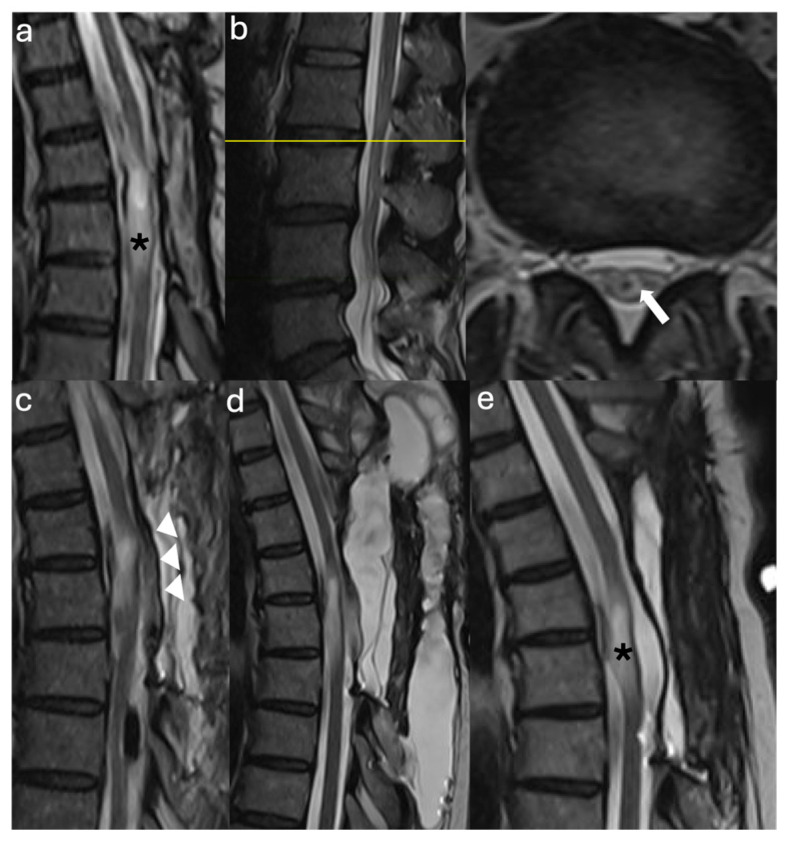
Radiographic Images Case 5: (a) Preoperative sagittal thoracic MRI T2 sequence shows syringohydromyelia associated with myelomalacia (asterisk) extending from T4–T6 at the prior T3–T5 laminectomy site for prior syrinx decompression. (b) Preoperative sagittal and axial lumbar MRI T2 sequences show a low-lying conus at the mid-L3 vertebral level. The conus tip is seen at the axial sequence at the upper L3 level (yellow bar, arrow). (c) Early postoperative sagittal thoracic MRI T2 sequence shows a significantly smaller thoracic syrinx with stable myelomalacia, expansile duraplasty (arrowheads). (d) One-month postoperative sagittal thoracic MRI T2 sequence shows a dorsal T3–T6, 4.7 × 3.4 × 11.1 cm sized pseudomeningocele. (e) Sagittal thoracic MRI T2 sequence after two duraplasty revisions shows no residual tethering and patulous CSF space posterior to the previously tethered spinal cord along with near resolution of the syrinx with residual pre-existing myelomalacia (asterisk).
